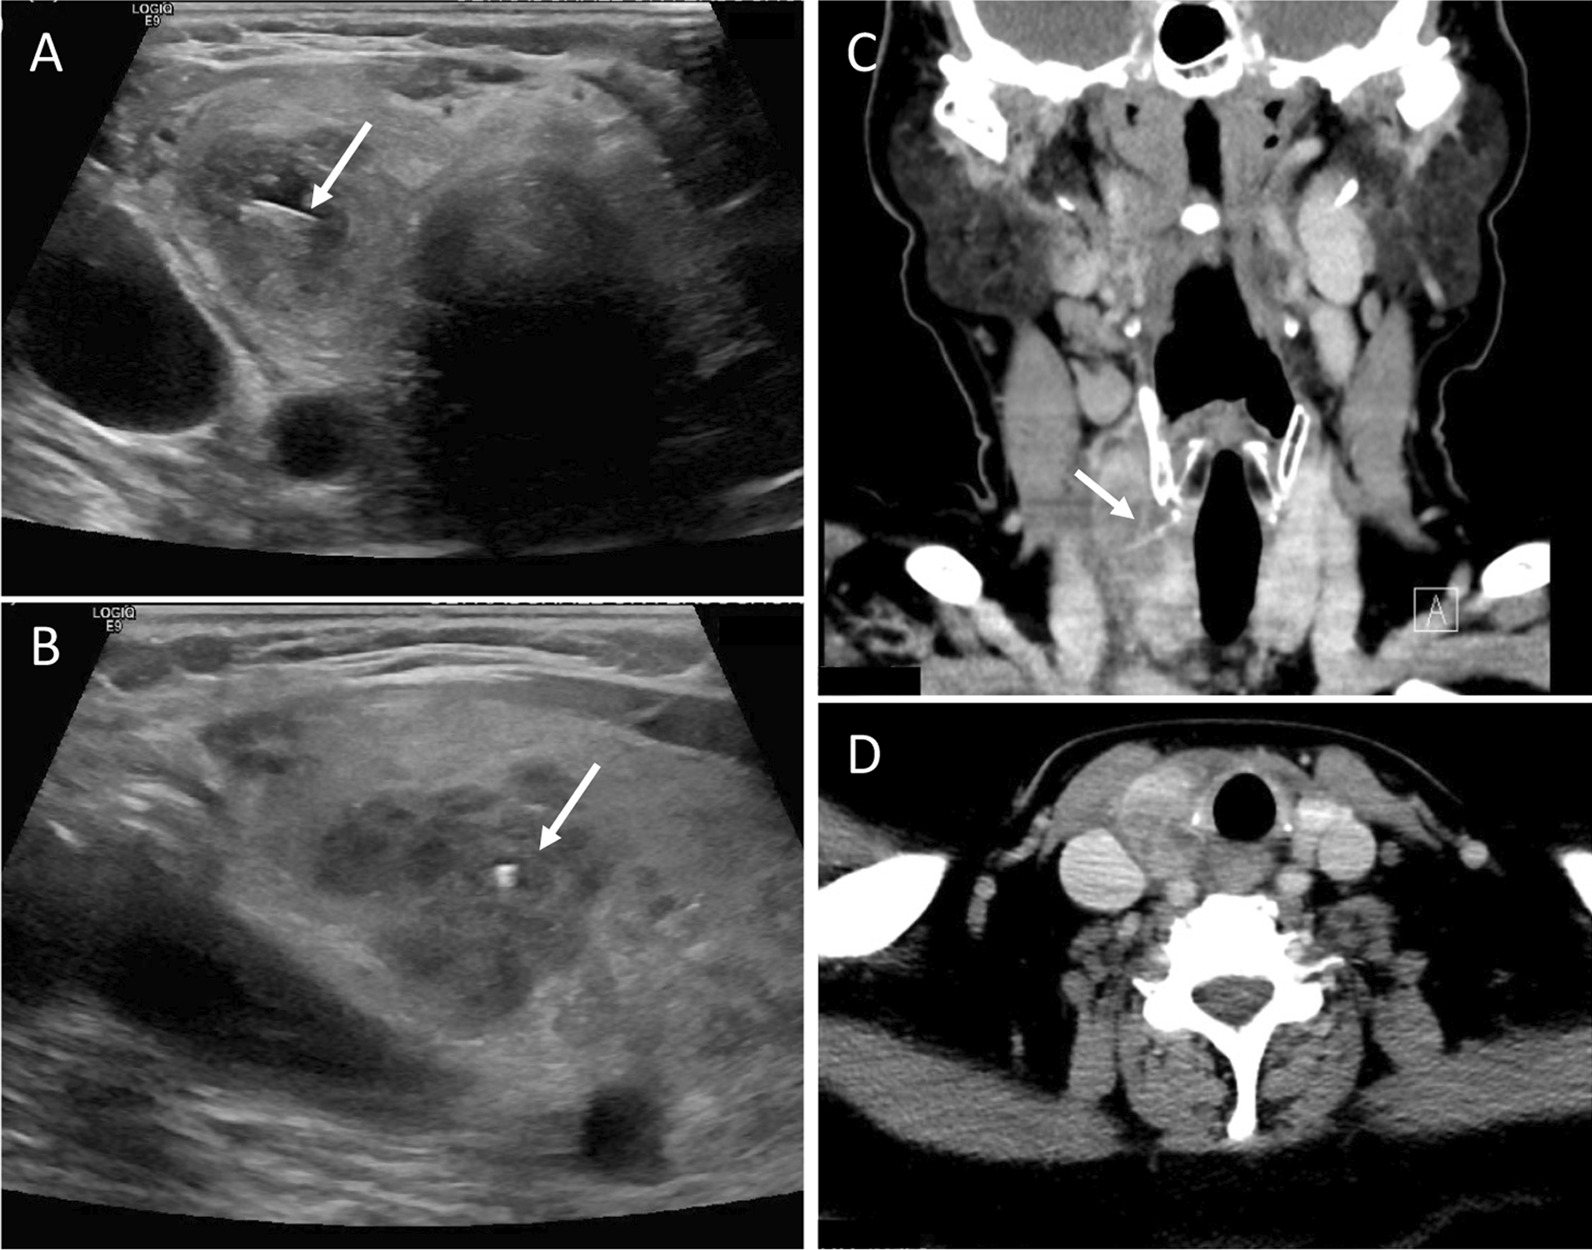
Diagnostic imaging of the neck. A and B CT scan of head and neck showing an inhomogeneous, swollen right thyroid lobe with a hypodense lesion (2.2 cm) as indicated by the arrows and inflammatory reaction of surrounding tissue. The left thyroid lobe appears unaltered with a normal size. No abscess was detected. A A thin hyperdense structure is present in the right thyroid lobe that was later identified to be a fish bone. C and D Ultrasound diagnostic confirmed the diagnosis of an enlarged thyroid lobe with a volume of 28.6 ml compared to 9.2 ml of the left thyroid lobe. A central, circumscribed area was identified in the right thyroid lobe, mainly hyperechoic with hypoechoic fractions and paranodular homogenous thyroid tissue without increase of perfusion. Within the inhomogeneous area, a sharp foreign body was identified. This was suspected to be a fish bone (arrows)
